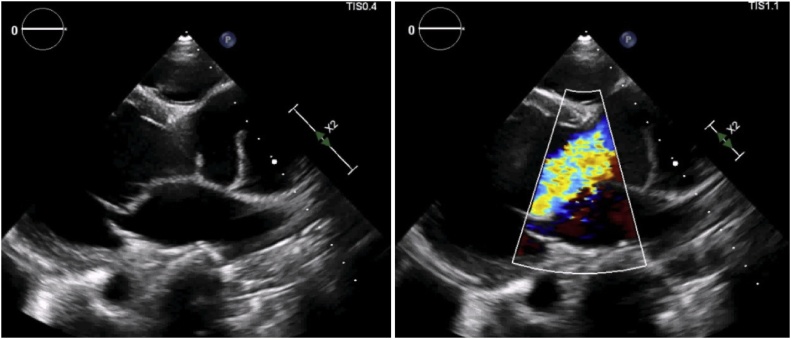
A: Parasternal long axis showing dilated aortic root & dilated LV. B: Color Doppler shows severe Aortic Regurgitation.
